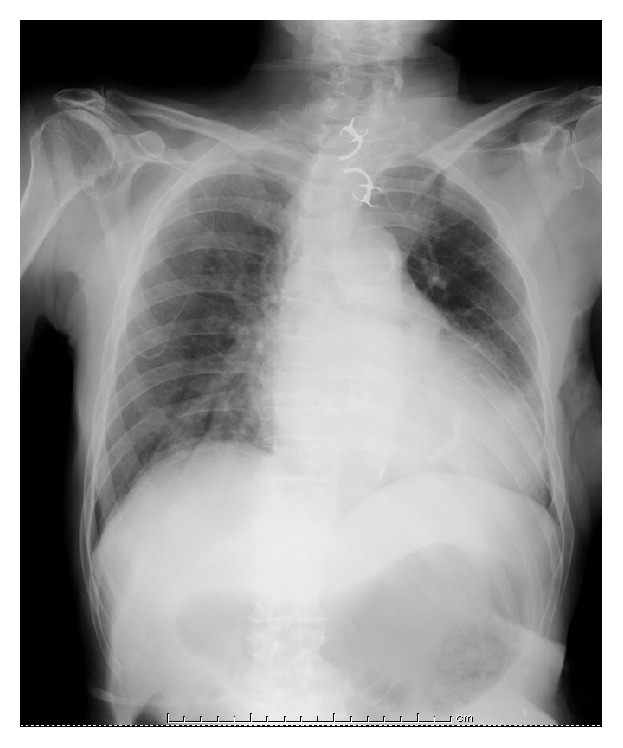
Plain chest radiography showing the ingested partial denture in the cervical esophagus.
